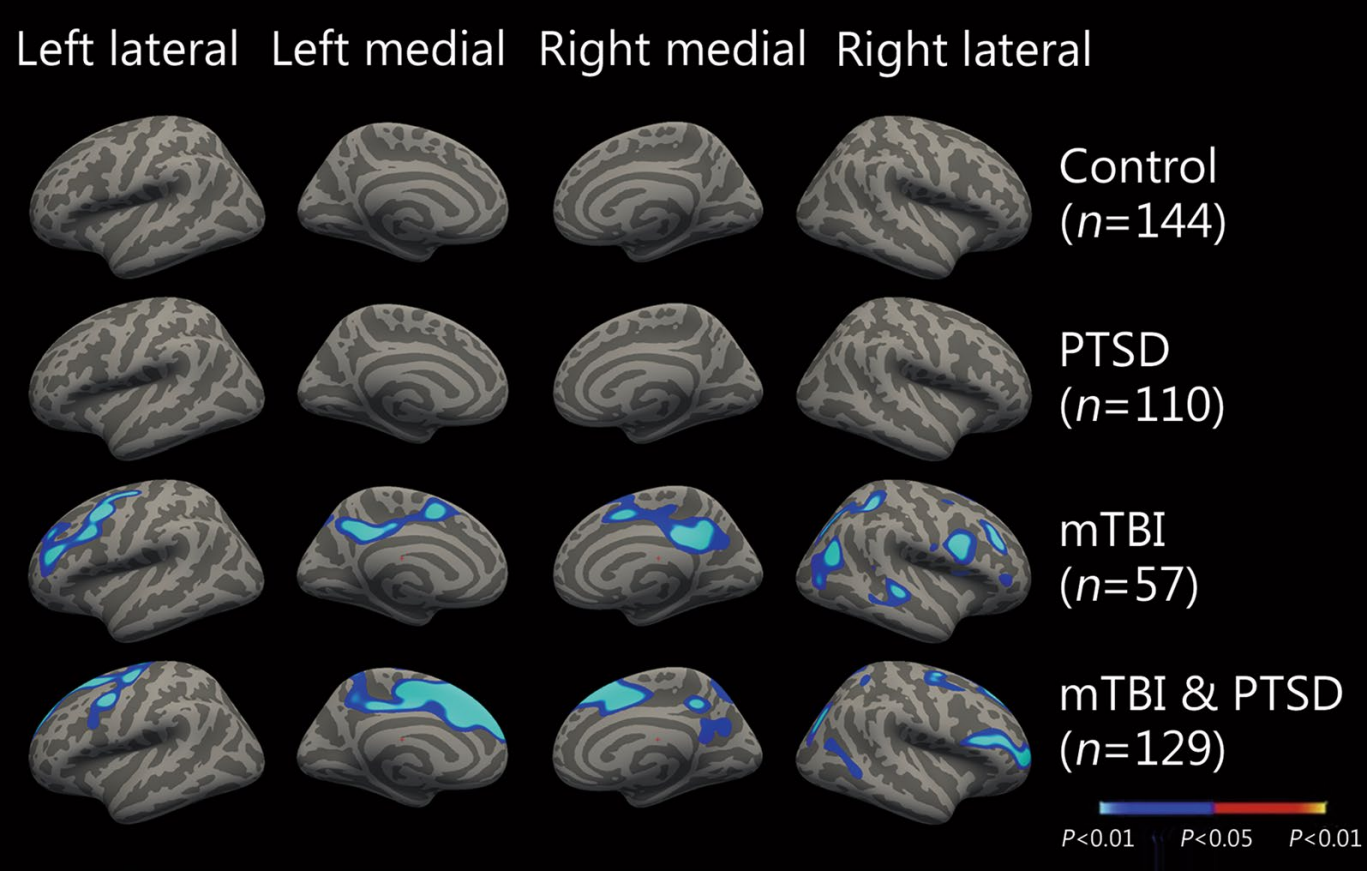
Magnetic resonance imaging (MRI) maps showing association of sleep with cortical thickness. Regional Associations between Pittsburgh Sleep Quality Index (PSQI) and cortical thickness in individuals with post-traumatic stress disorder (PTSD, *n* = 110), mild traumatic brain injury (mTBI, *n* = 57), both diagnoses (comorbid mTBI and PTSD, *n* = 129), and neither diagnosis (neither mTBI nor PTSD, *n* = 144). Blue indicates regions in which increased PSQI (poorer sleep) was significantly associated with decreased cortical thickness
